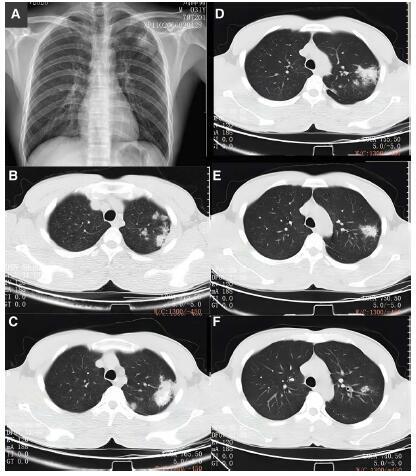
Chest X-ray of the patient on November 6, 2020 and Chest CT of the patient taken on November 9, 2020.
